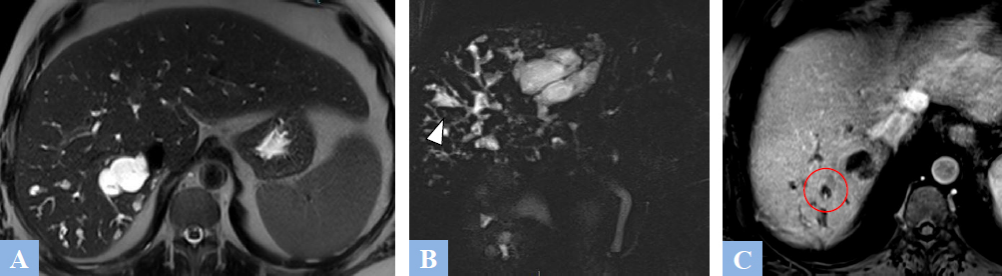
Examples of biliary hamartomatosis. A. T2-weighted MR image in the axial plane. Numerous subcentimeter cystic lesions with T2 hyperintensity across all hepatic segments. B. 2D radial MR cholangiography showing countless millimetric hepatic cystic lesions creating a “starry sky” appearance. C. 2D radial MR cholangiography. Multiple larger hepatic cystic lesions are consistent with biliary hamartomas.
